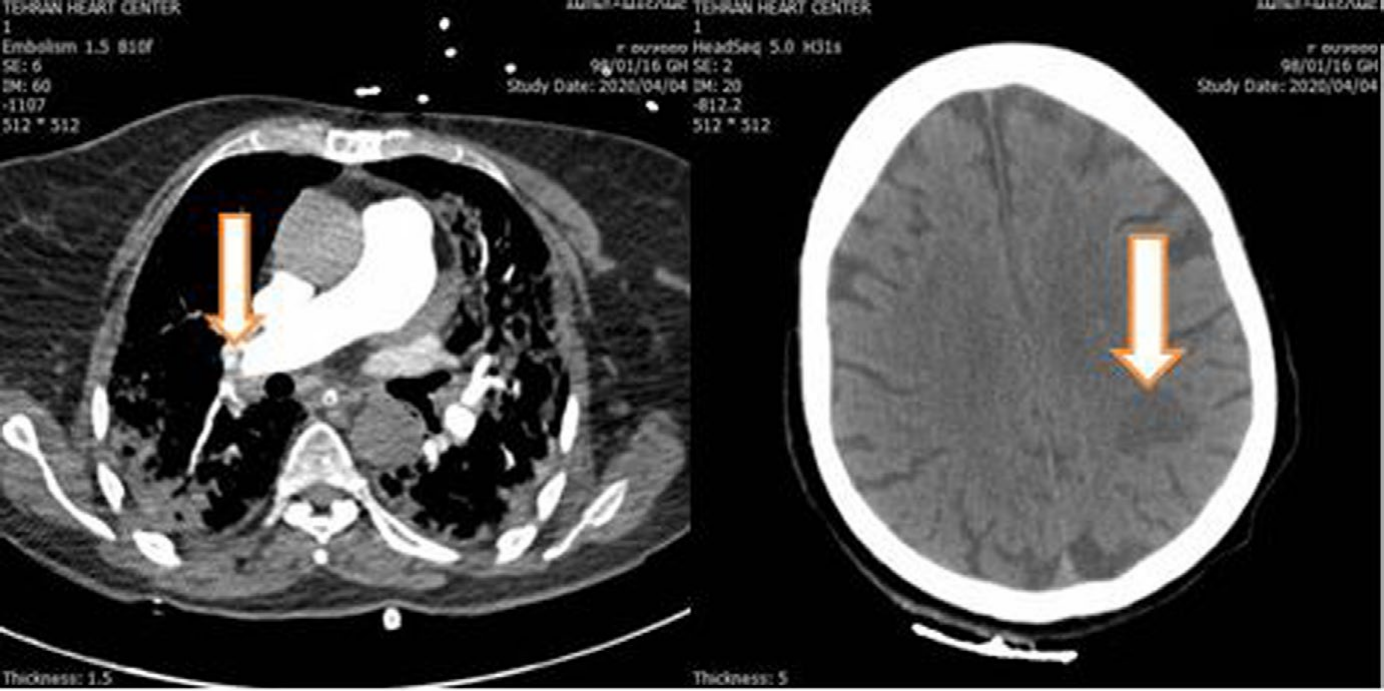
Left panel: Pulmonary thromboembolism in right lobar pulmonary artery, white arrow. Right panel: Brain CT. Probable thromboembolic ischemia in left parieto‐occipital area, white arrow
